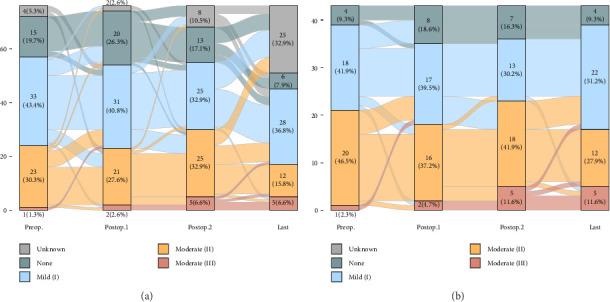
The changes in the grading of AR. No patient showed progression to severe AR during the follow-up. (a) Results with missing data across the four time points (*n* = 76). (b) Complete sets of echocardiographic data at all four time points (*n* = 43). Mean follow-up interval: Postop.1: 10.6 ± 4.8 days; Postop.2: 17.9 ± 2.4 months; Last: 6.3 ± 0.5 years. The terminology “Preop.” refers to the assessment conducted just before MV surgery, “Postop.1” refers to the initial echocardiographic findings following MV surgery, “Postop.2” refers to results approximately one year after MV surgery, and “Last” designates the most recent echocardiographic assessment conducted prior to the AV intervention, aside from the one-year follow-up. In cases where the one-year results were the latest available, they were treated as the “Postop.1” assessment.
